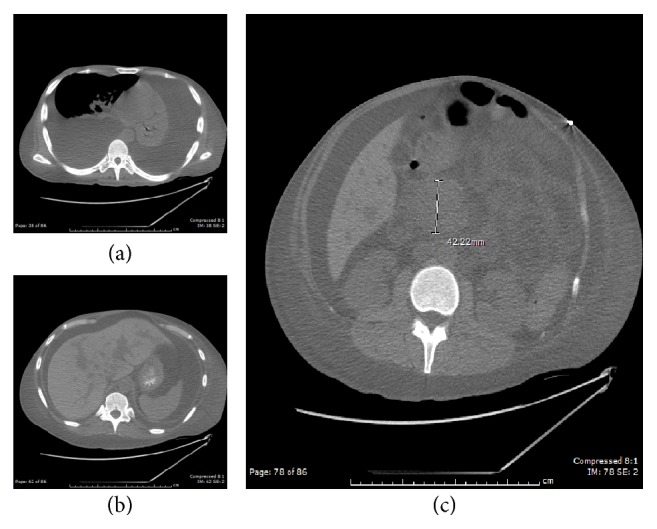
CT scans of the chest and abdomen show large bilateral pleural effusions (a) and severe ascites (b). There is a 4.2 cm mass in the head of the pancreas (c).
